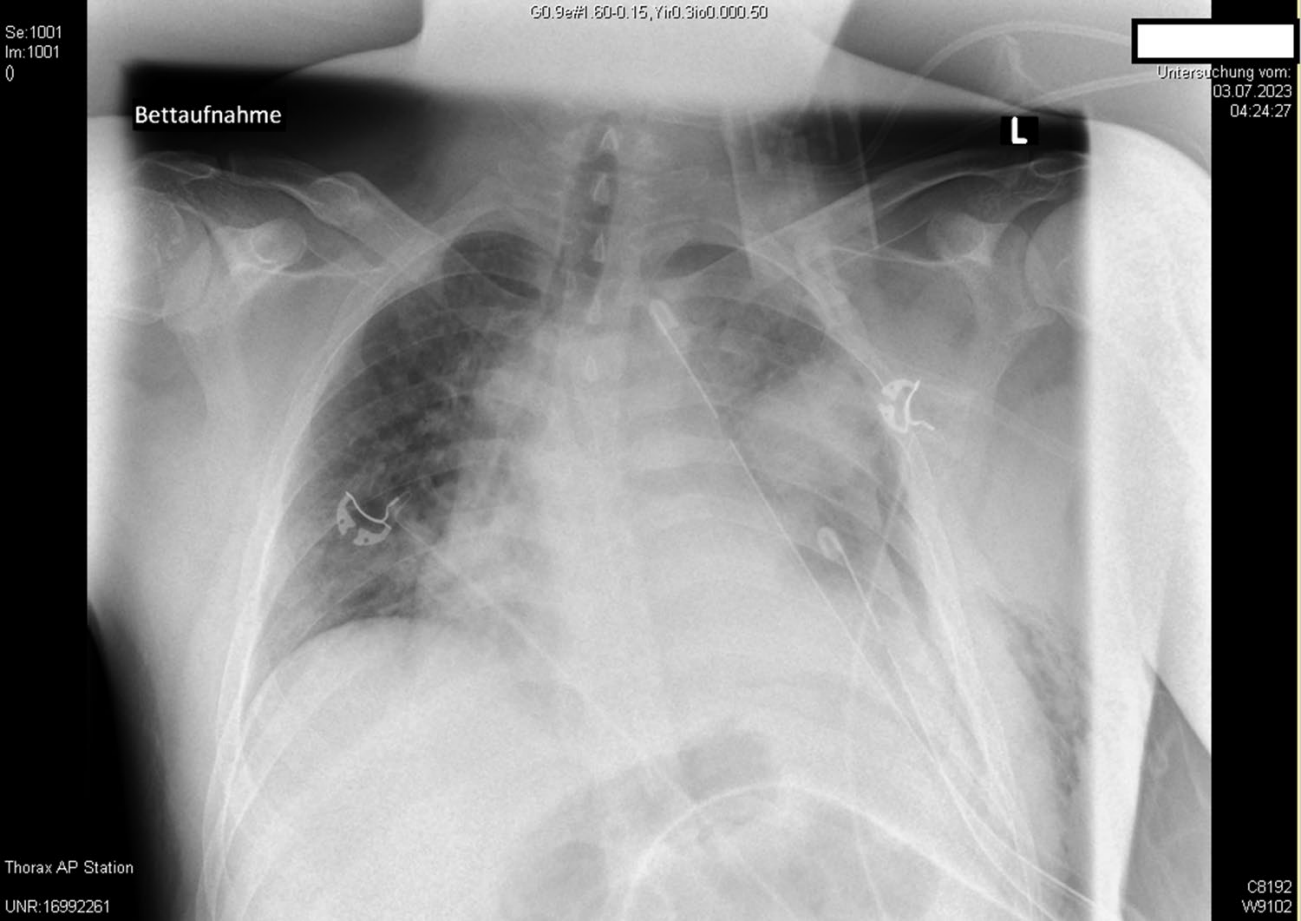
Chest radiography upon ICU admission, with two indwelling left sided chest drains in place, in the presence of persistent left sided pneumo- and hemothorax
